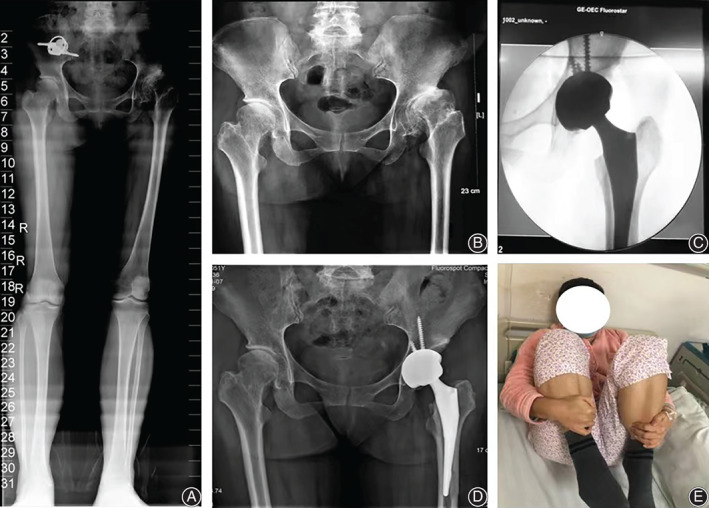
The 73rd case on the learning curve with unilateral hip dysplasia treated with DA‐THA. (A) Preoperative full‐length radiograph of lower extremity. (B) Preoperative anteroposterior radiograph of pelvis. (C) Intraoperative radiograph. (D) Postoperative anteroposterior radiograph of pelvis in the last follow‐up for 13 months. (E)The hip activity on the first postoperative day
